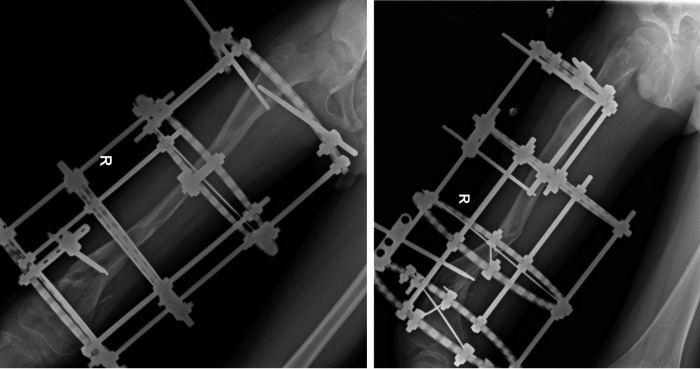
Taken 7 months postoperatively, shows a right lateral view x-ray of the femur, indicating fracture healing, followed by the removal of the external fixation device.
